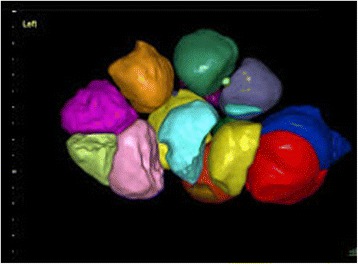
3D ultrasound’s image to determine antral follicle count. Legend: Ultrasound image by 3D SonoAVC’s technique that allows counting the number of antral follicles by individual colors determined by the software. Their volume can also be determined based on the anechoic intrafollicular areas in the scanner that is automatically calculated
